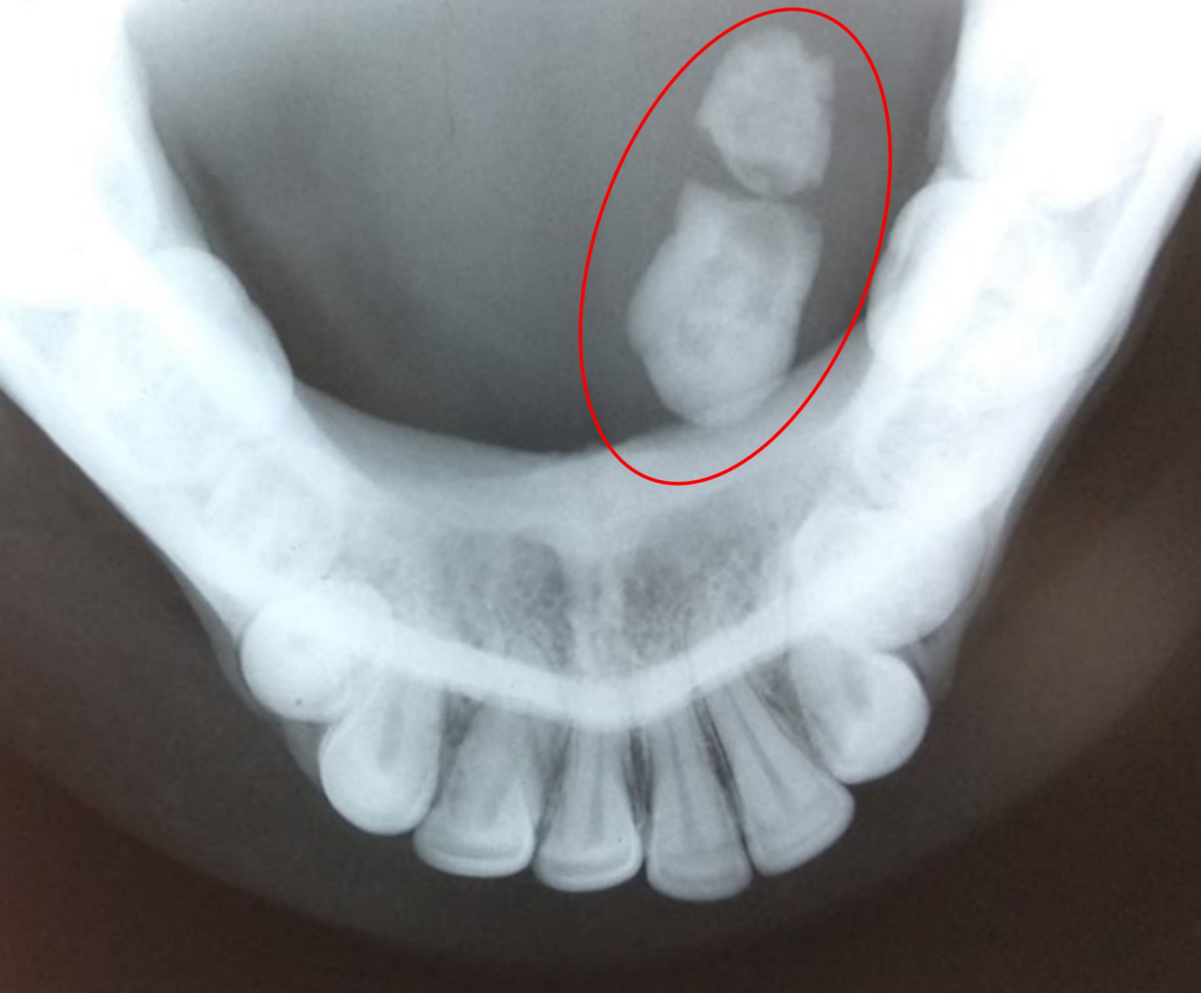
Occlusal radiograph showing salivary gland calculi
